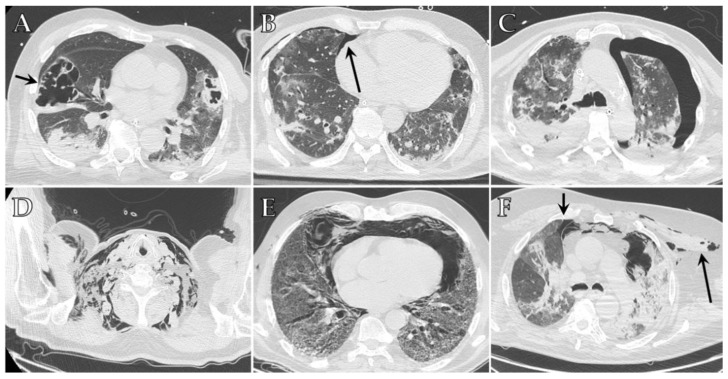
Axial HRCT images of different patients with COVID-19 ARDS admitted to ICU showing imaging features indicative of barotrauma secondary to mechanical ventilation: (A) large pneumatocele (black arrow); (B) small anterior pneumothorax (black arrow); (C) large left pneumothorax; (D) extensive bilateral subcutaneous emphysema of the neck; (E) pneumomediastinum; (F) pneumomediastinum associated with small anterior pneumothorax (small black arrow) and subcutaneous emphysema of the left upper chest (long black arrow).
